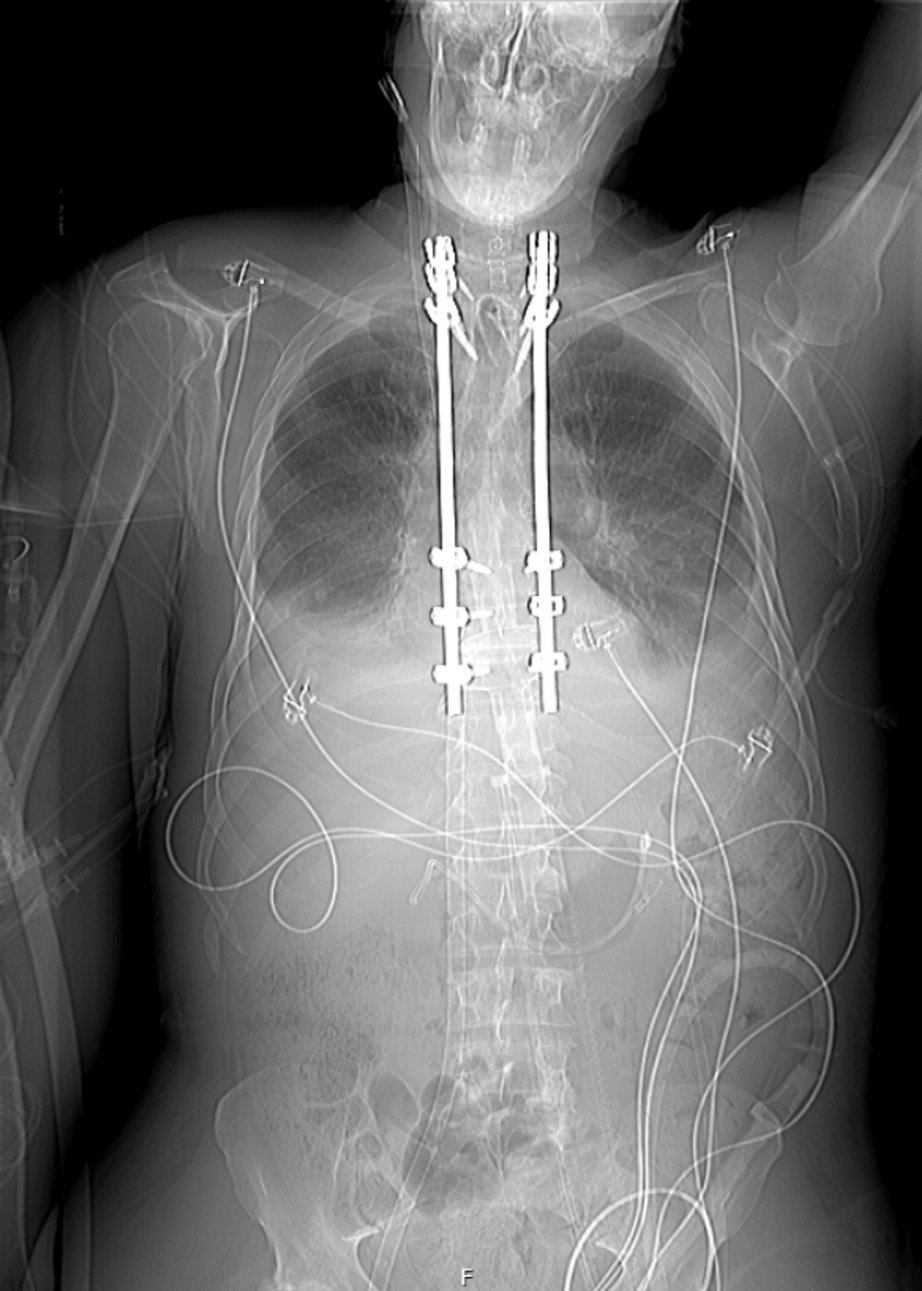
The patient's post-operative X-ray
